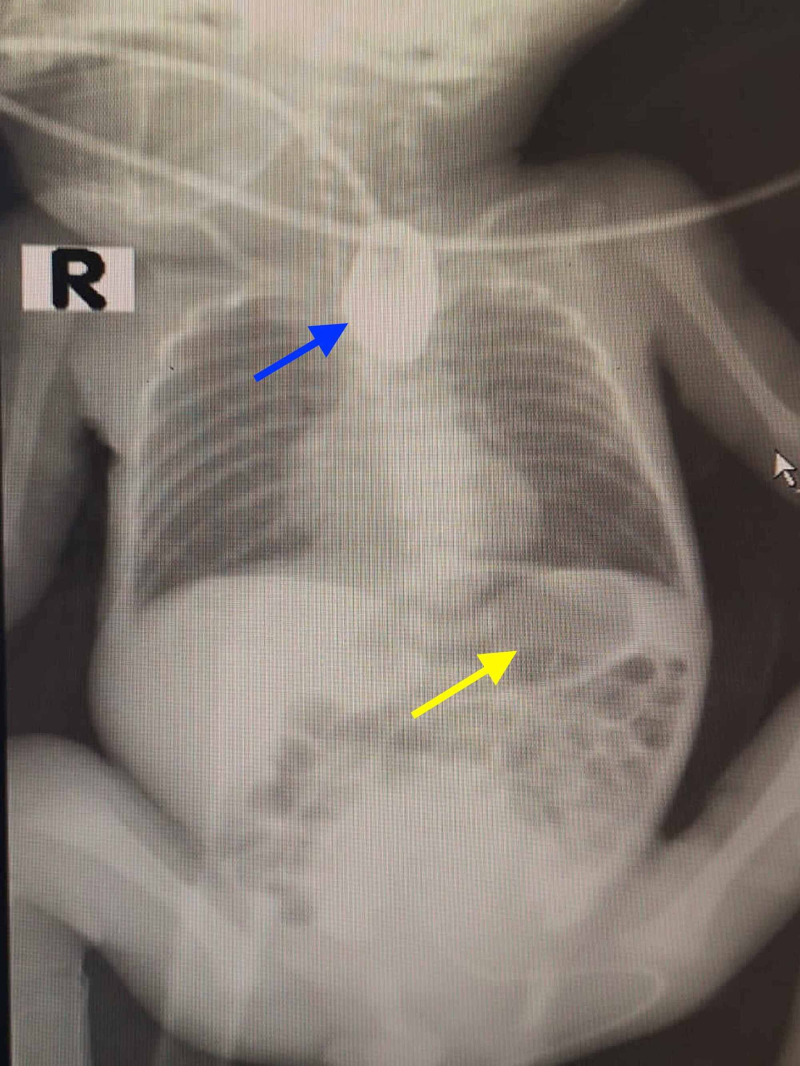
A preoperative radiograph showing the presence of contrast material within the upper esophageal pouch (blue arrow). The presence of gas in the stomach is also noted (yellow arrow).
